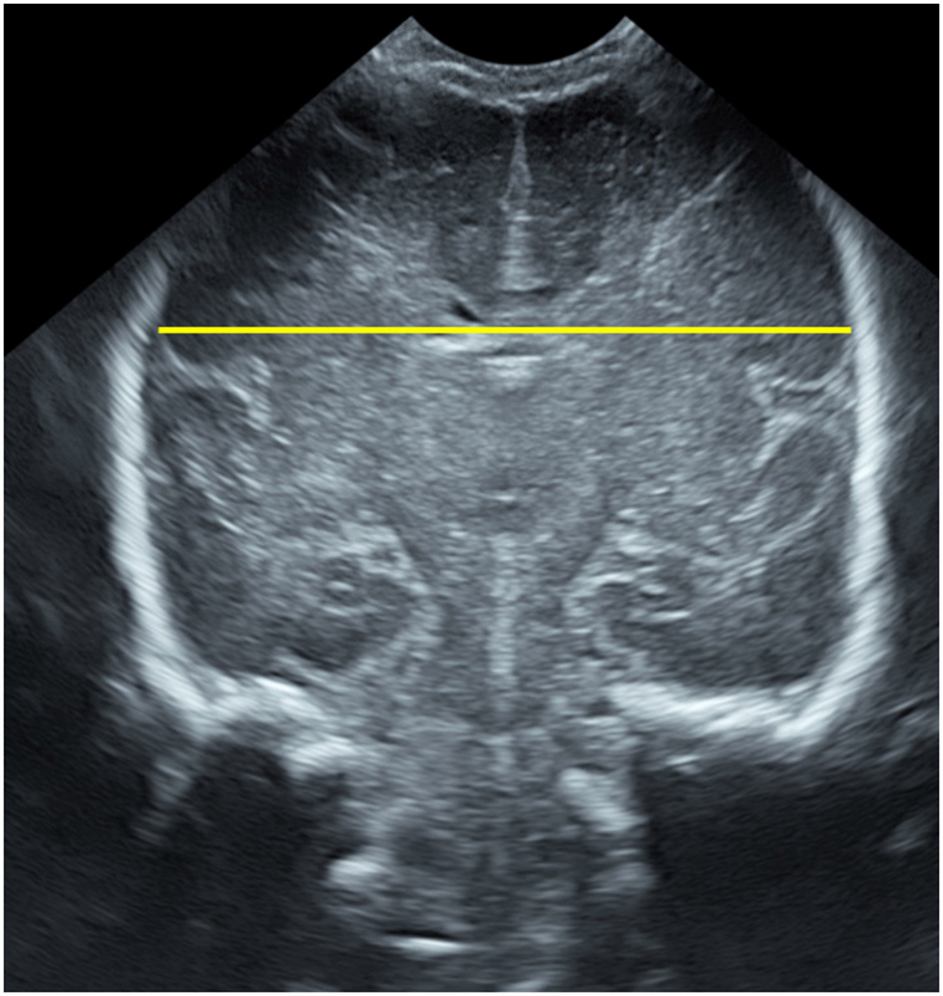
Biparietal diameter measured on the ultrasonographic third coronal plane.
